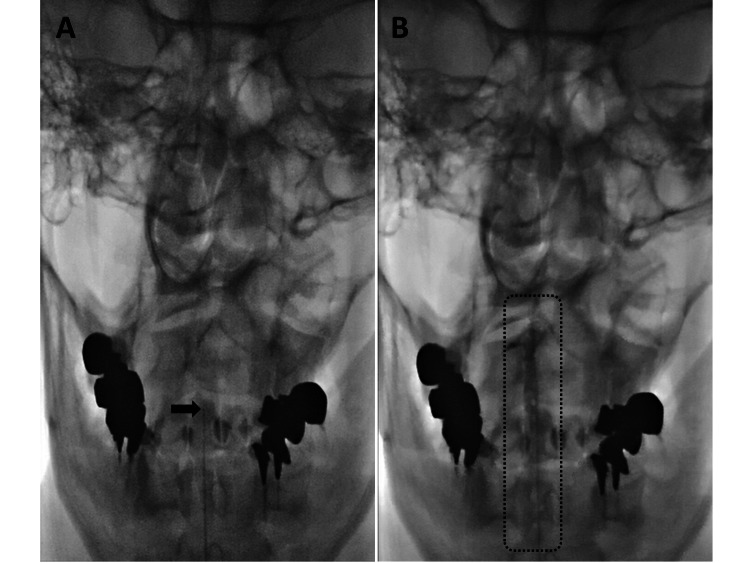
X-ray image. X-ray image of the front before (A) and after injection of blood and contrast agent (B). The black arrow indicates the tip of the Racz catheter (A). The dotted-line rectangle indicates the contrast agent (B).
